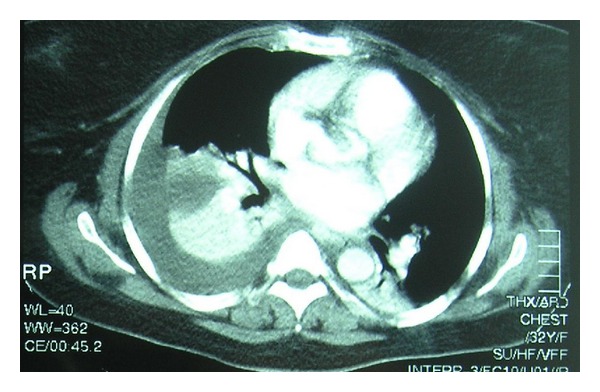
Case 2: CT scan revealing ipsilateral pleural effusion.
